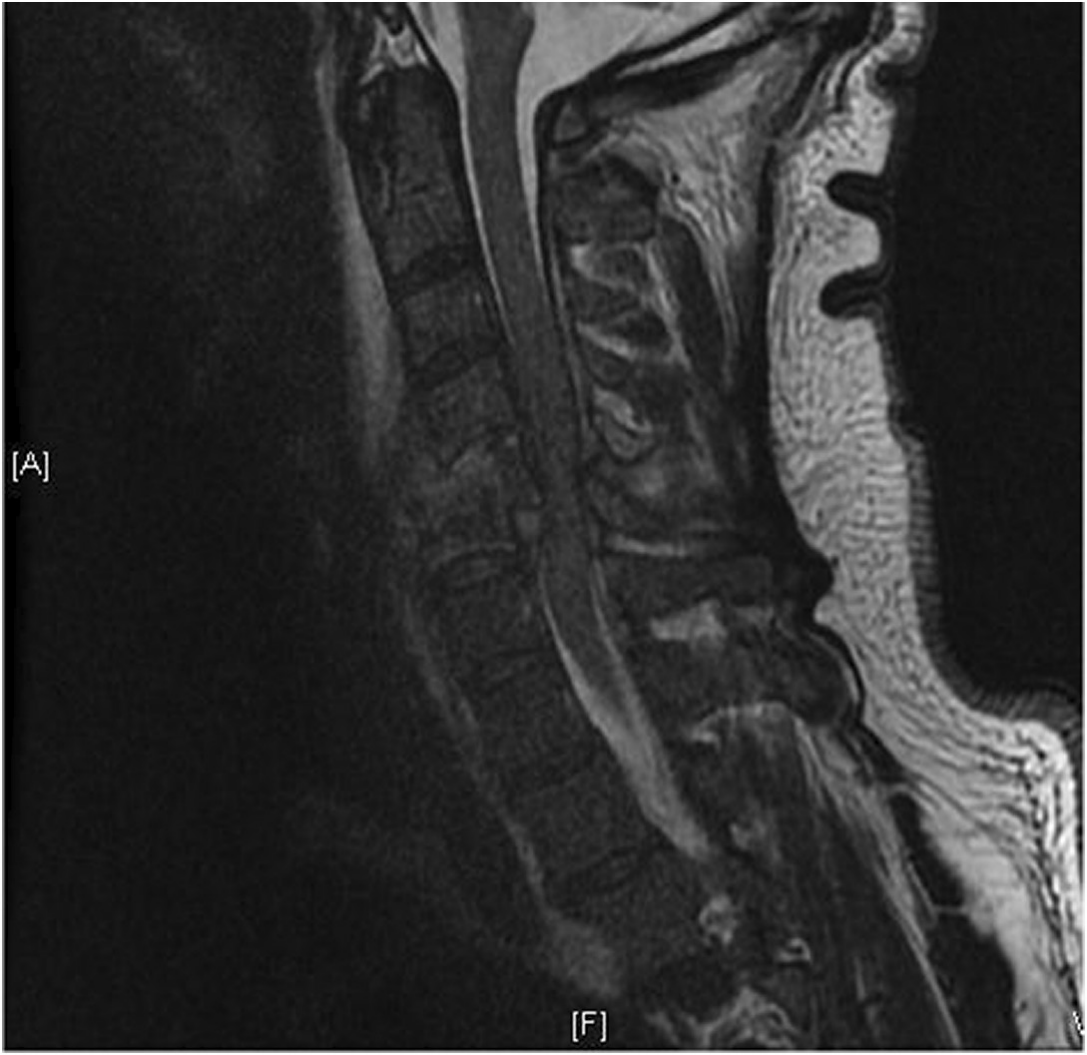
Sagittal MRI view showing diskitis/osteomyelitis at C4‐5 and C5‐6, with the collapse of the C5 vertebral body. Multilevel epidural phlegmon/abscess resulting in severe spinal stenosis and moderate cord compression from C4‐C6. Extensive prevertebral and paravertebral phlegmon/abscess is also seen.
